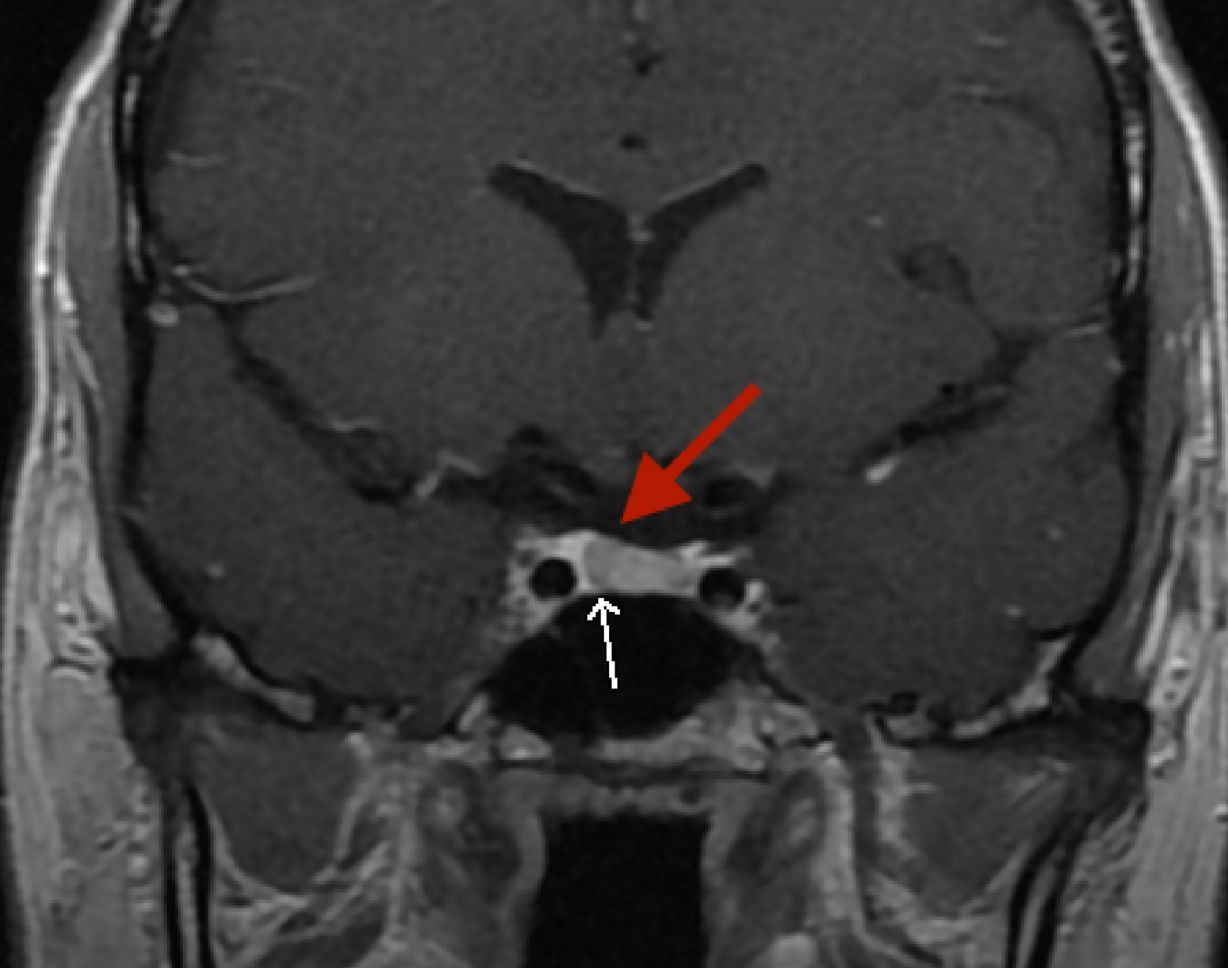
Gadolinium-enhanced T1-weighted coronal MRI: subtle right pituitary hypo-enhancement (white arrow) with elevation of diaphragmatic sella (red arrow) MRI: Magnetic Resonance Imaging
